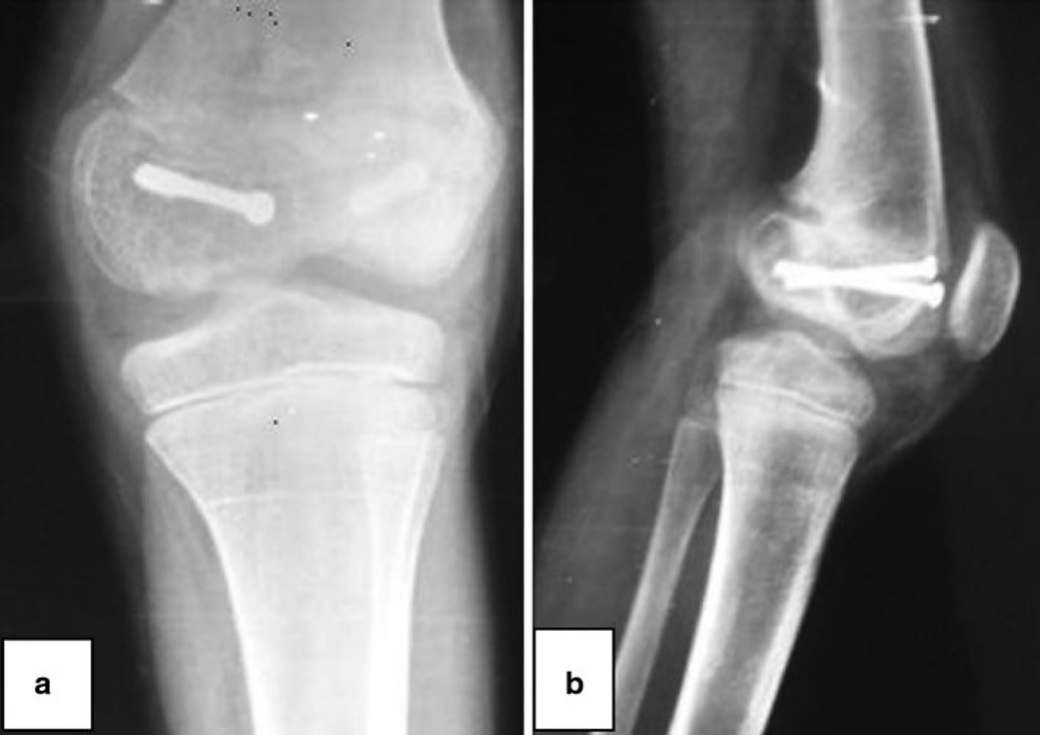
Final follow-up anteroposterior a and lateral b radiographs, showing anatomical union of the fracture site with no attendant osteoarthritic or avascular changes in the knee joint
